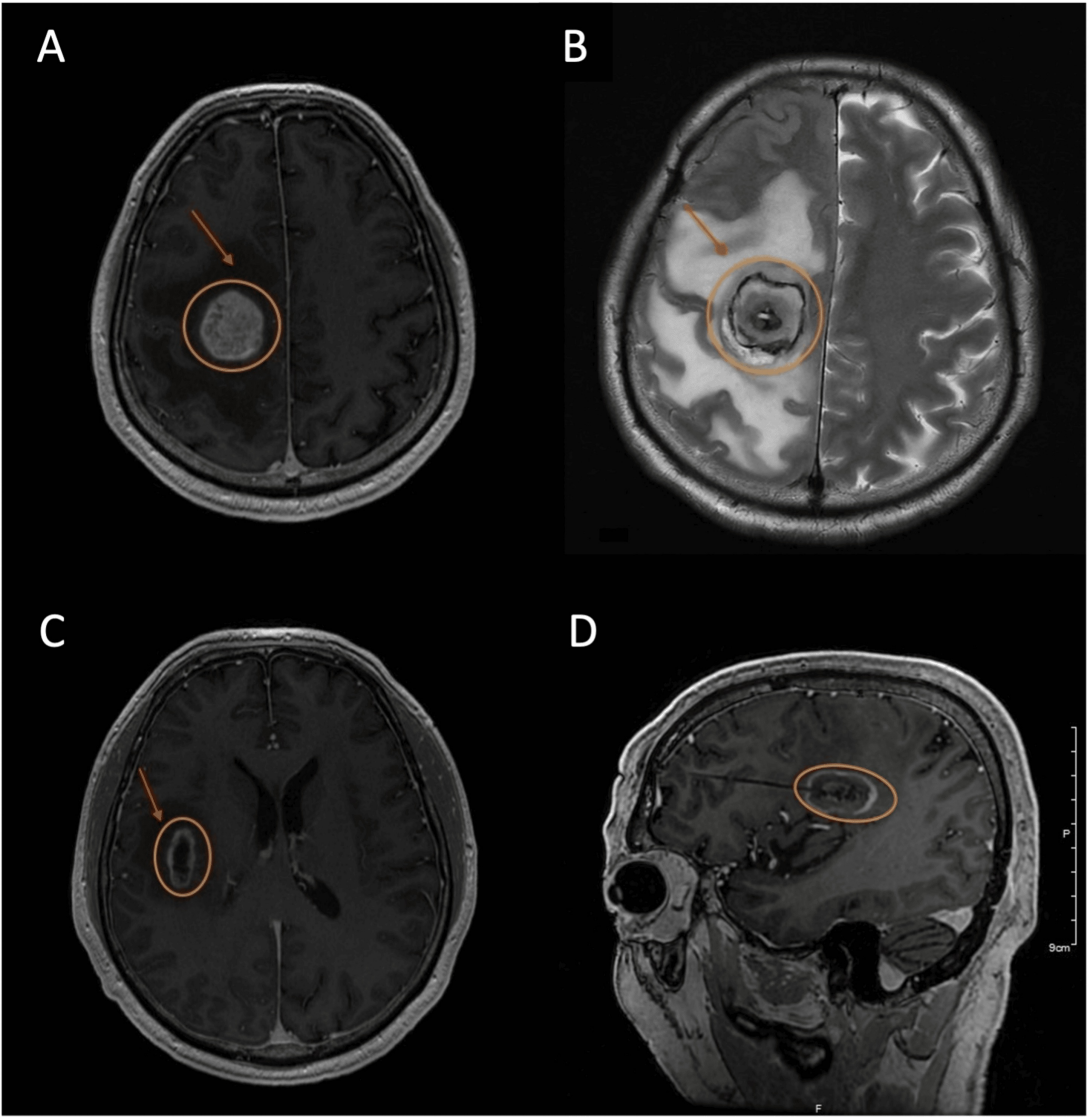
MRI scans pre- and post-operative (A) pre-operative axial T1 FSPGR + contrast MRI; (B) axial T2 FSE ACS MRI 24 hours post-operative of the right frontal lobe large GBM (orange), surrounded by edema (red arrow); (C) pre-operative axial T1 FSPGR + contrast MRI; (D) sagittal T1 + contrast MRI 24 hours post-operative of the smaller tumor necrosis (orange) and edema (red arrow)
